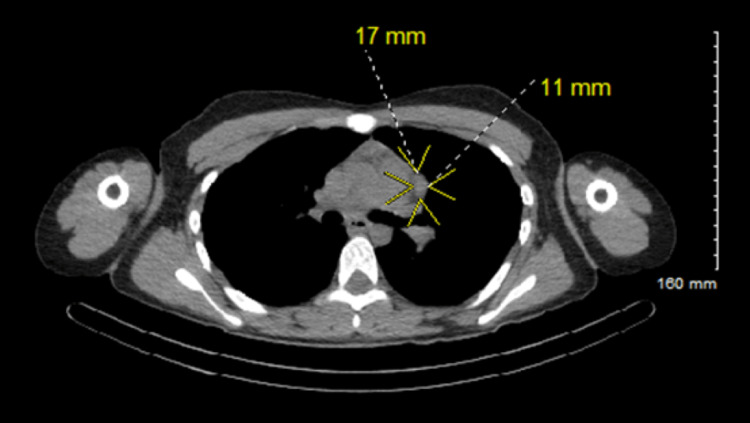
Confirmatory CT imaging of prevascular mediastinal parathyroid adenoma High-quality CT scan without contrast of the chest with isolation (yellow arrows) and orthogonal measurements based on the longest primary axis of the ovoid 17 mm x 11 mm mediastinal lesion (yellow-colored font adjacent to white-dashed line).
